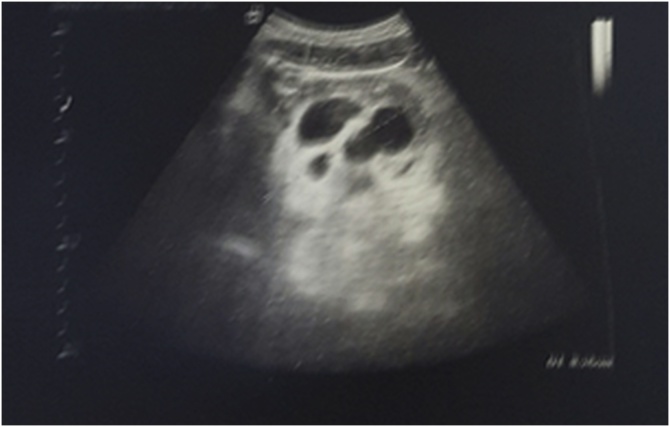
An ultrasound picture showing a 10*7 cm multiloculated thick walled lesion.
